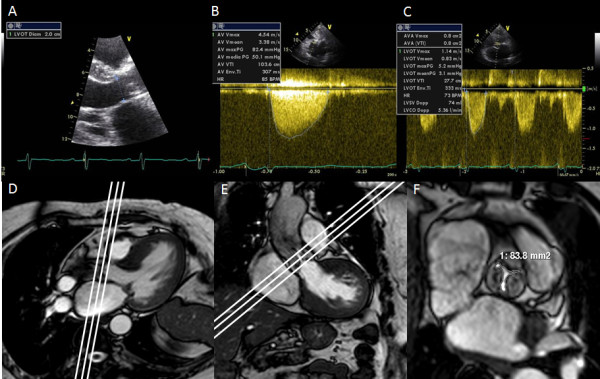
Aortic valve area measurement by trans-thoracic echocardiography (TTE) and cardiovascular magnetic resonance (CMR). Aortic valve area measured by continuity equation TTE approach: Left ventricular outflow tract (LVOT) diameter (A); transaortic peak velocity (B); aortic velocity-time integral (C). Oblique sagittal view of the aortic outflow tract, with the CMR slice position indicated by three white lines orthogonal to the stenotic jet (D). Oblique transaxial view of the aortic outflow tract, with CMR slice position indicated by three white lines (E). Cross-sectional view of a severely stenotic aortic valve; the white line denotes the aortic valve area (F).
